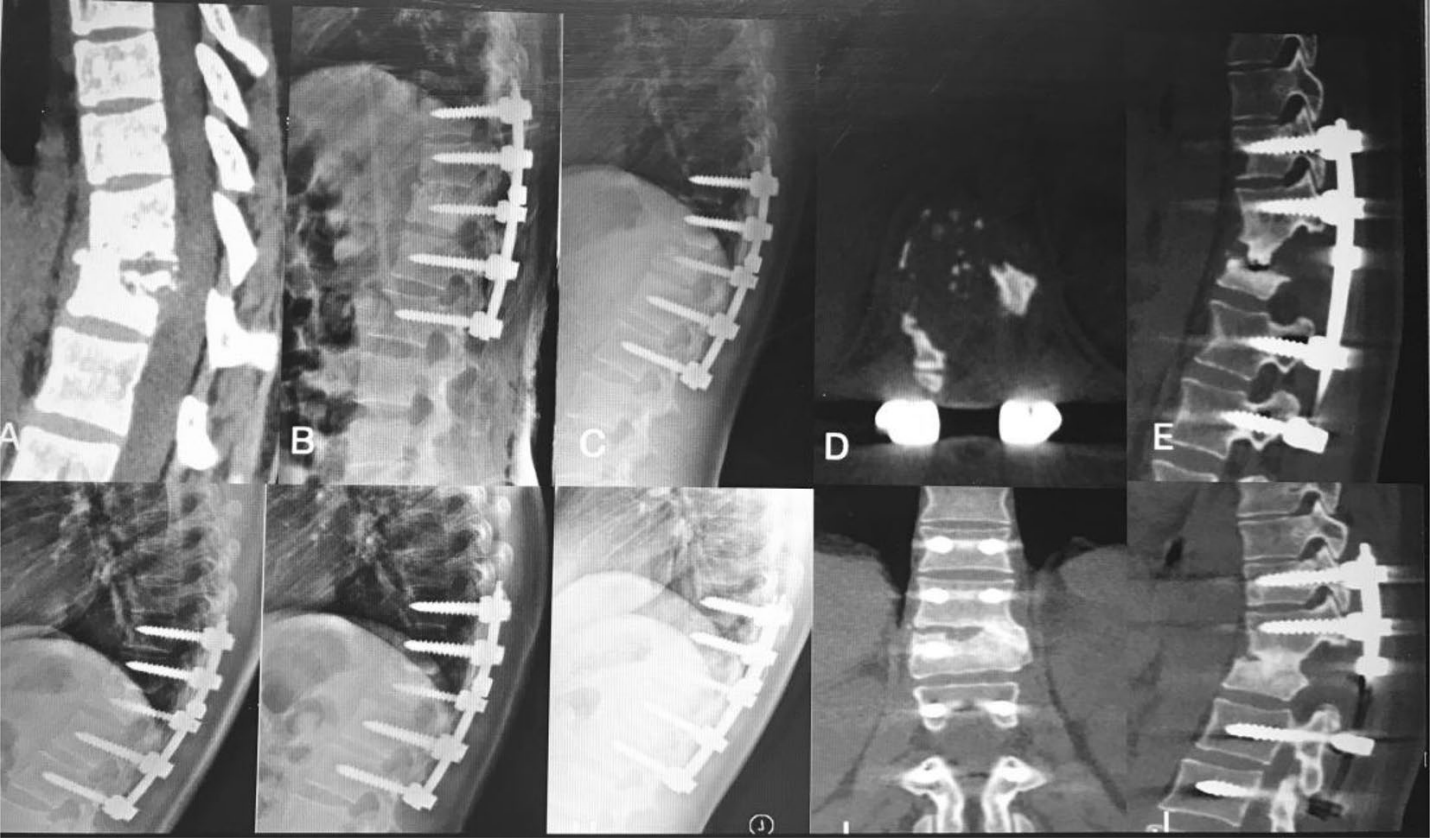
A 22-year-old female patient with thoracic spinal tuberculosis (T11–12) underwent posterior debridement and decompression combined with instrumentation. (A) Preoperative computed tomography (CT) shows bone destruction of the T11–12 vertebrae and compression of the spinal cord. (B–E) The 1-week and 6-month postoperative X-rays and 6-month postoperative CT show the correction was maintained, but the bone was not fully healed. (F,G) The 1-year and 2-year postoperative X-ray show the kyphosis correction had been lost. (H–J) At 5-year FU, plain X-ray and CT showed solid bone fusion.
